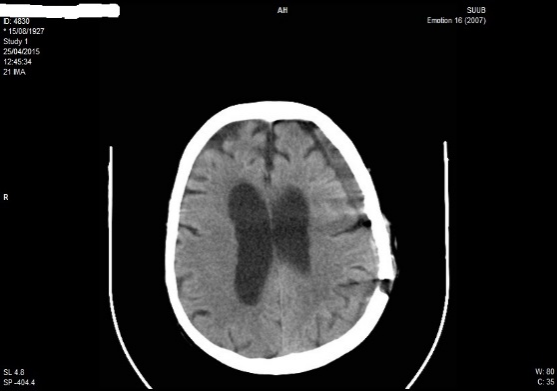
CT scan at 5 days postoperatory in patient P.M. of 87 years old; a little left F-T restant serohematic line can be observed, without a compressive effect on the brain
